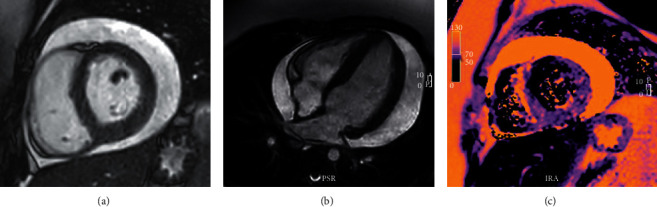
Cardiac MRI, cine short-axis (a) and 4-chamber (b) views showing a moderate-sized pericardial effusion. (c) Short-axis T2 map showing myocardial T2 is elevated (70 ms) consistent with diffuse edema.
